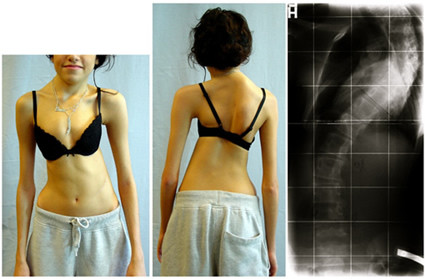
Patient with failure of segmentation without decompensation due to conservative treatment. This 18 years old girl presented at the age of 10, with progressive congenital scoliosis with rib synostosis due to failure of segmentation. The patient has denied surgery. During the last follow - up, the clinical appearance demonstrates that a severe decompensation as had to be expected has been prevented. The radiograph demonstrates a curve of 72°. VC was 650 ml, 19% of the predicted value.
